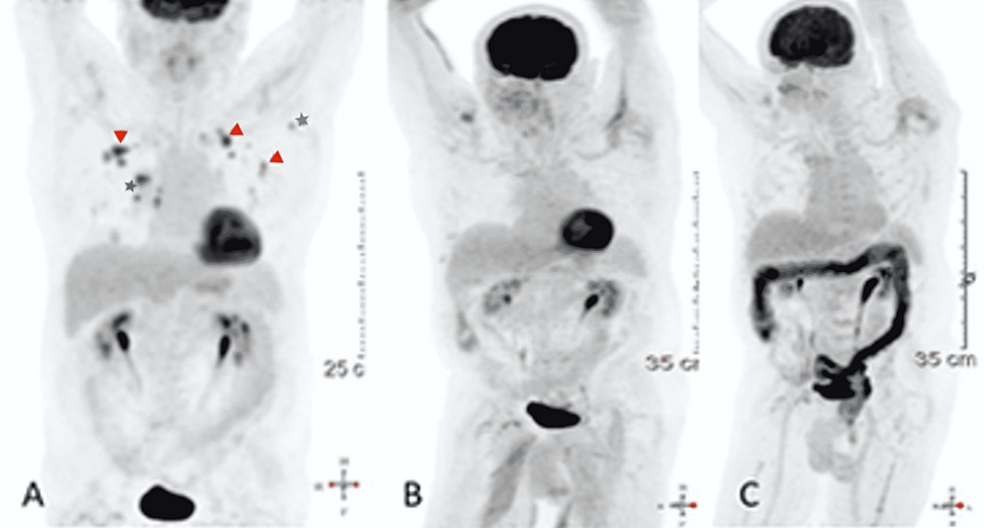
PET scan after cycles 15 and 16 of the adjusted regimen. A) Initial positron-emission tomography–computed tomography (PET/CT), maximum intensity projection (MIP), demonstrates multiple bilateral hypermetabolic pulmonary nodules, intense fluorodeoxyglucose (FDG) avid right hilar metastatic lymphadenopathy, and metastatic hypermetabolic left axillary lymph node (Red arrowhead: hypermetabolic pulmonary nodules, Green star: hilar metastatic lymphadenopathy, Blue star: hypermetabolic axillary lymphadenopathy). B) MIP image, after completion of five cycles of carboplatin/nab-paclitaxel/pembrolizumab. C) MIP images after 16 cycles of pembrolizumab show resolution of previous hypermetabolic activity of multiple bilateral pulmonary nodules, consistent with response to treatment; also incidentally showed diffuse inflammatory bowel activity.
